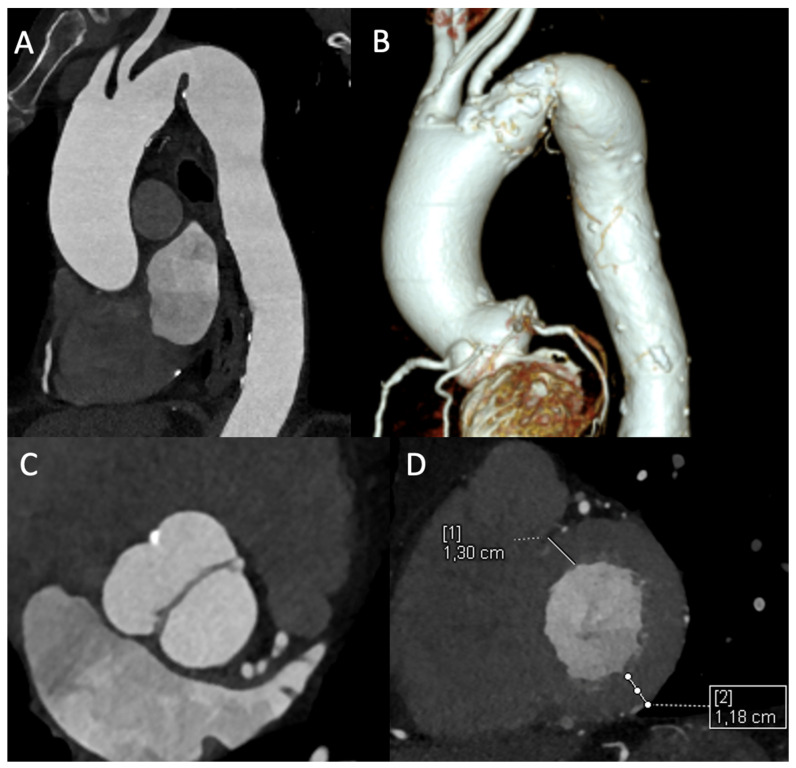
Assessment of aortic coarctation by CT. (A) CTA sagittal view of aortic coarctation; (B) CTA 3D volume rendering reconstruction of aortic coarctation; (C) CTA with detailed view of the bicuspid aortic valve in diastolic phase; (D) CTA with reconstruction on ventricular short-axis view and measurement of ventricular walls in diastolic phase for assessment of LV hypertrophy.
